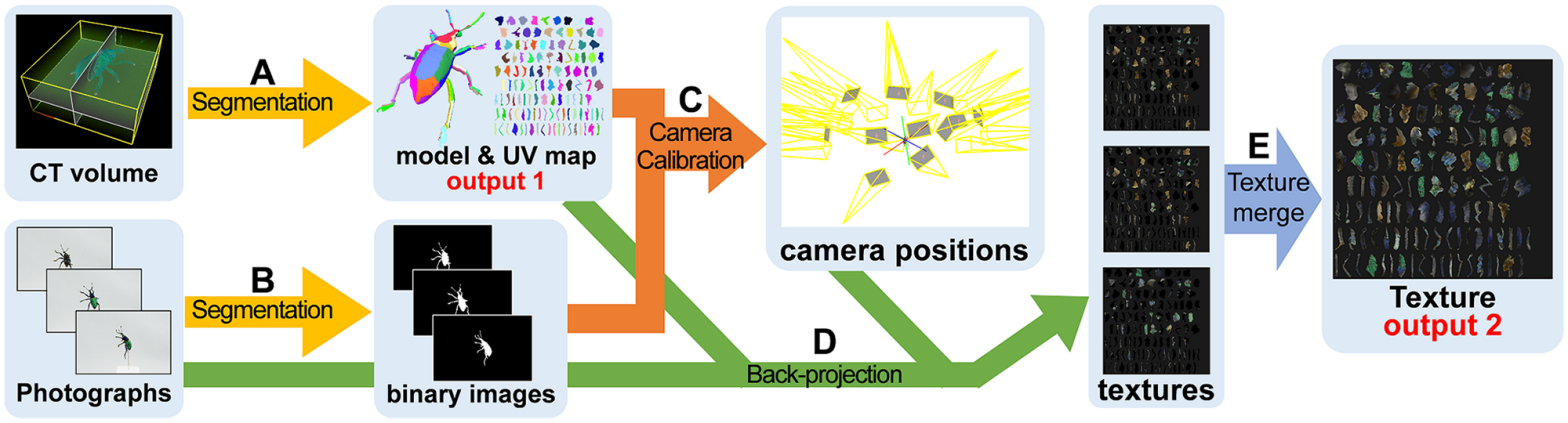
Overview of our digitization processes. Input CT volume is binarized to generate 3D model (A). Input photographs are also binarized (B). We estimate camera position relative to 3D model by using binarized photographs (C) and back project all photographs onto 3D model to obtain texture atlases (D). All texture atlases are merged to generate single texture (E).
